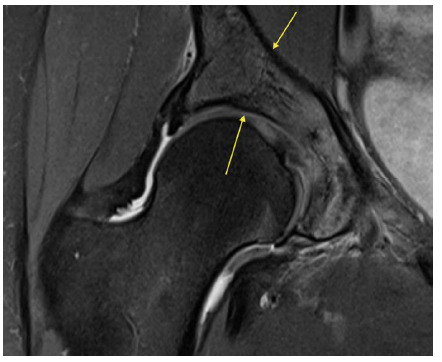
Case 2: MRI scan of the right hip on the day of injury demonstrating fracture of the acetabular roof
